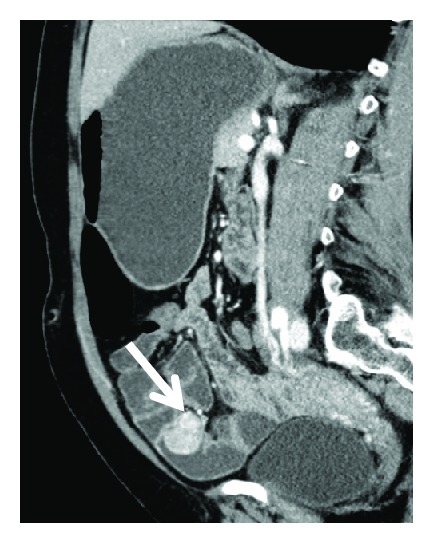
CT enterography—sagittal CT image with lesion compatible with GIST.
